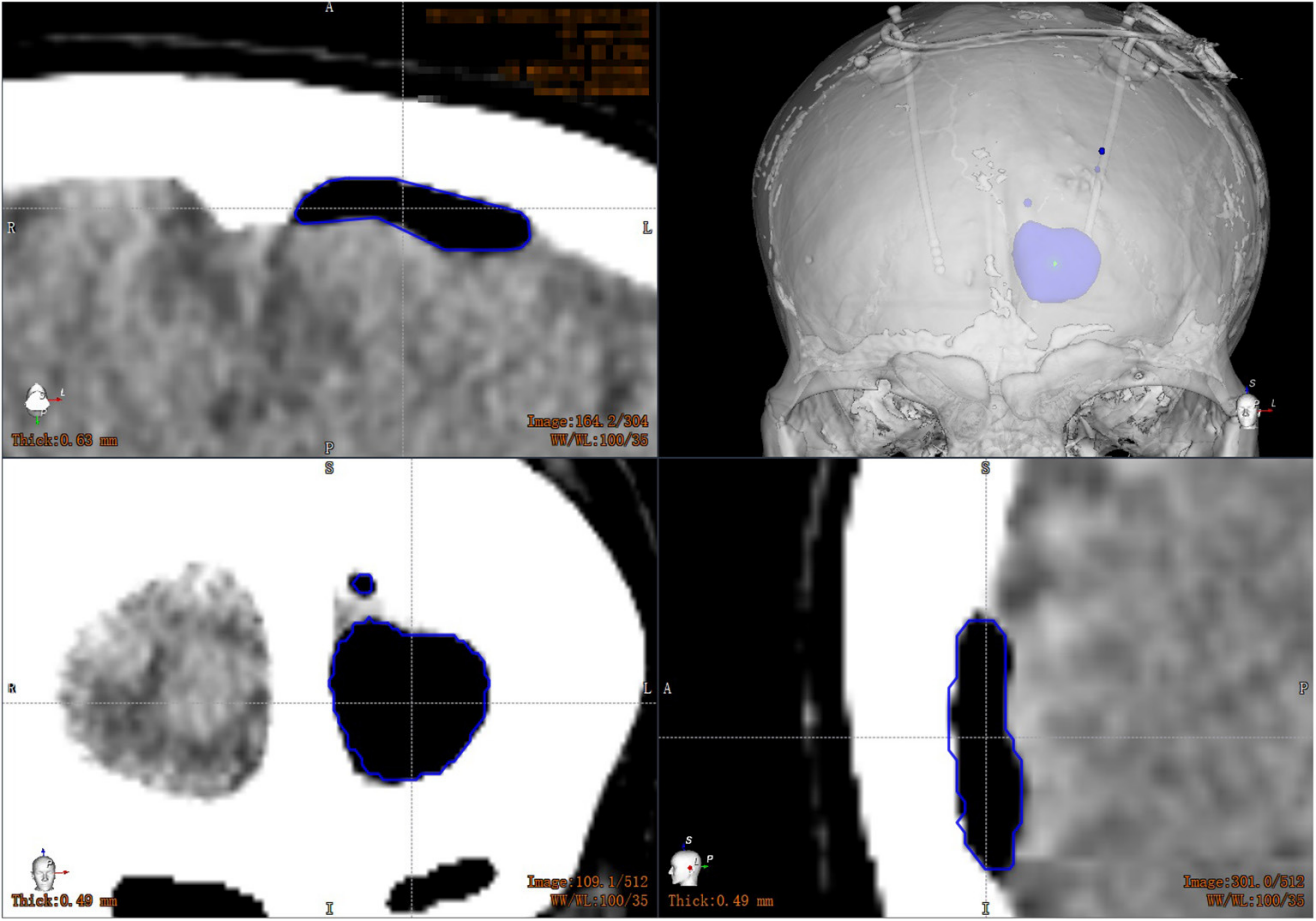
Volume calculation of intracranial air: the CT images are transmitted to the workstation, and the computer automatically calculates the volume of the patient's intracranial air layer by layer.
